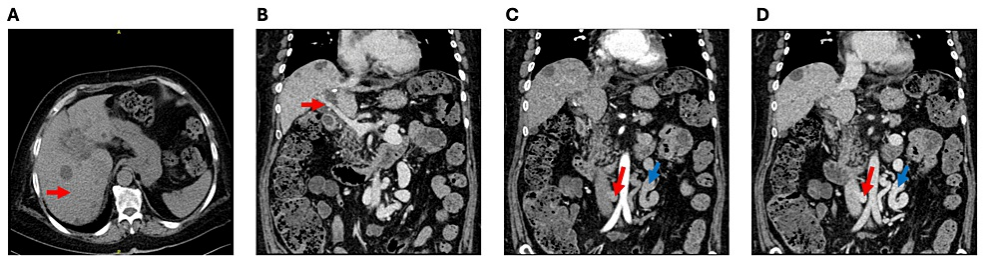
Contrast-enhanced abdominal CT (A) Liver (axial view, non-contrast): the red arrow points to the cirrhotic liver. (B) Portal vein (coronal view, portal phase): the red arrow points to the narrow portal vein. (C) Collateral circulation (coronal view, arterial phase): the red arrow points to the wide collateral vein, and the blue arrow points to other parts of the collateral vein. (D) Collateral circulation (coronal view, portal phase): the red arrow points to the wide collateral vein, and the blue arrow points to other parts of the collateral vein.
